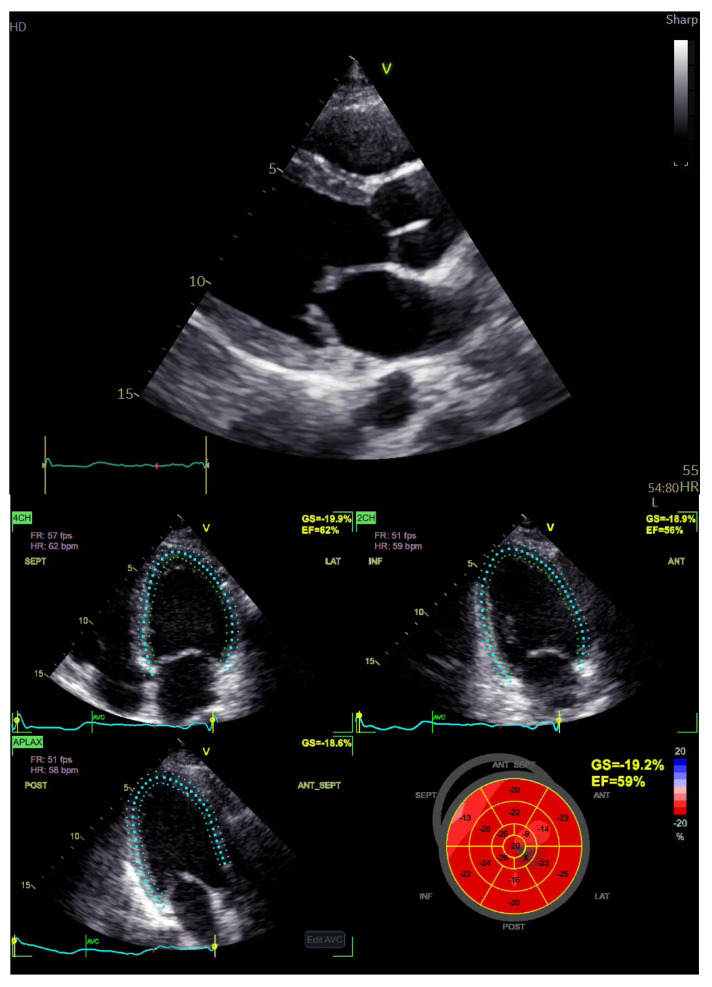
Transthoracic echocardiography findings in the patient, showing normal left-ventricular dimensions and contractility; upper panel, parasternal short-axis view; lower panel, left-ventricular ejection fraction and global longitudinal strain estimations. GS, global strain; EF, ejection fraction; 4CH, 4−chamber view; APLAX, apical long axis; 2CH, 2−chamber view; HR, heart rate; FR, frequency; SEPT, septal; LAT, lateral; INF, inferior; ANT, anterior; POST, posterior; ANT_SEPT, anteroseptal.
